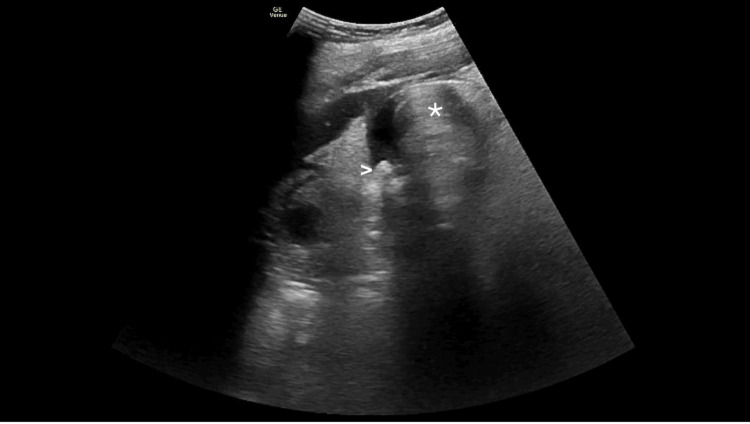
Right upper quadrant point-of-care ultrasonography showing shadowing gallstones (arrowhead) and prominent gas shadowing (*) originating from within the nondependent fundus and obscuring most of the gallbladder and posterior wall.
